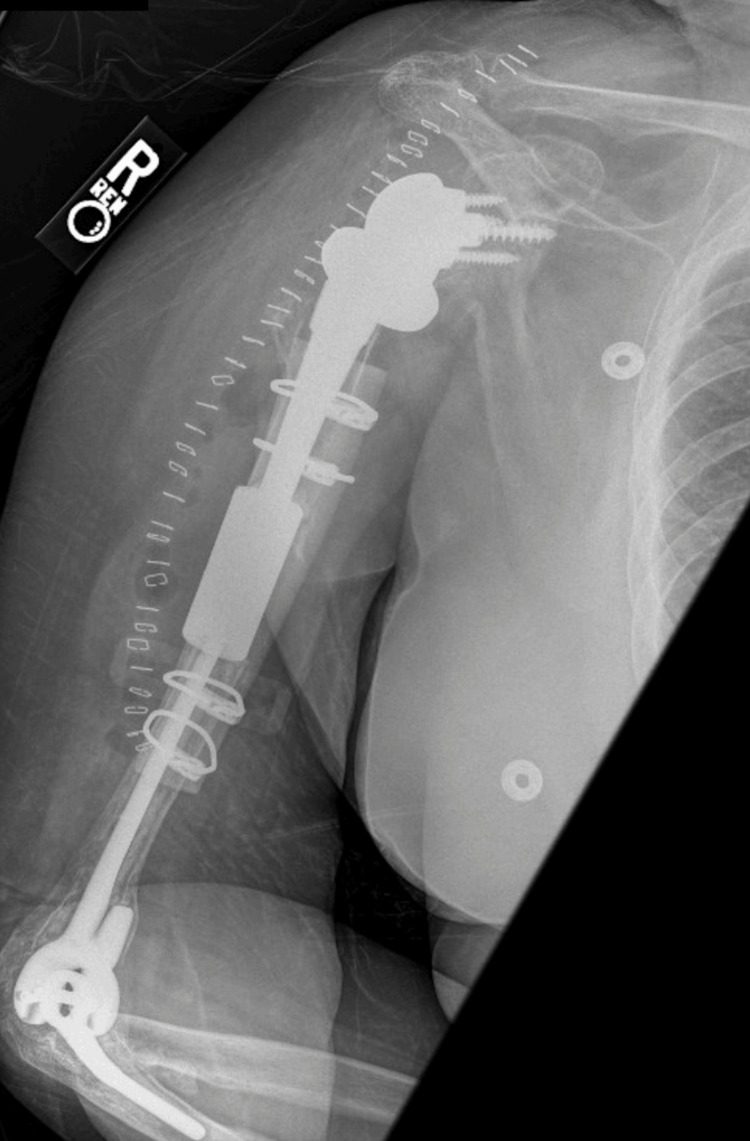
POD0 lateral radiograph of the right humerus with allograft repair and RTSA. POD: postoperative day; RTSA: reverse total shoulder arthroplasty
